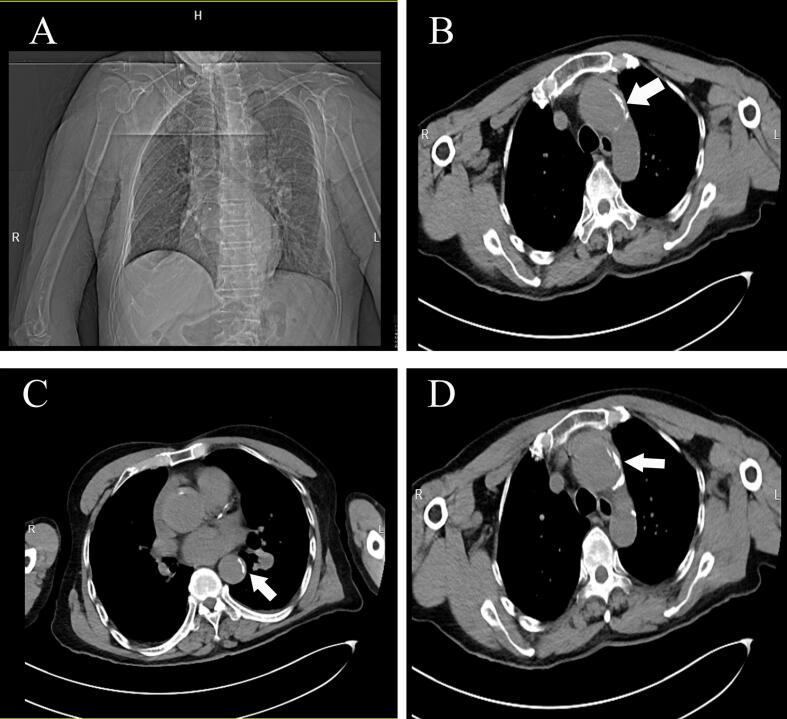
Non-contrast chest computed tomography (CT) scan shows eccentric wall thickening with hyper-density (“crescent sign”) (white arrows).
